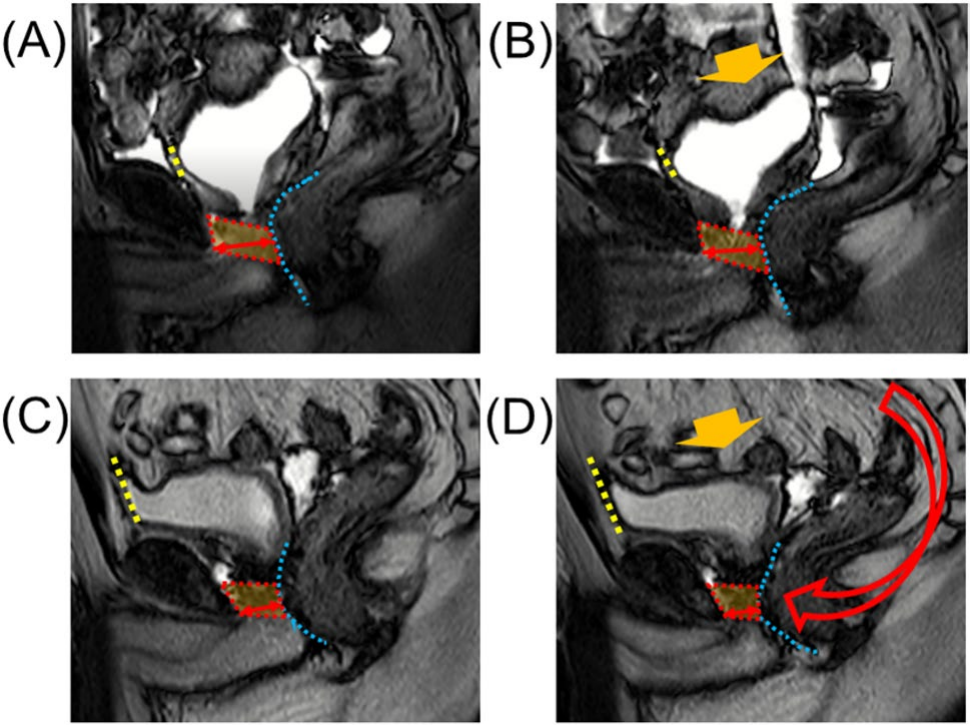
Dynamic mid-sagittal magnetic resonance imaging (MRI) after conventional robot-assisted radical prostatectomy (RARP): at rest (A) and with abdominal pressure (B). Dynamic mid-sagittal MRI after Retzius-sparing RARP: at rest (C) and with abdominal pressure (D). The thickness of the external urethral sphincter (two-headed red arrow) defined as the distance from the lowest point of the pubic bone to the anterior edge of the rectal wall (blue dashed line) was measured at rest and with abdominal pressure (orange arrow). The external urethral sphincter is indicated by the box surrounded by the red dashed line.
